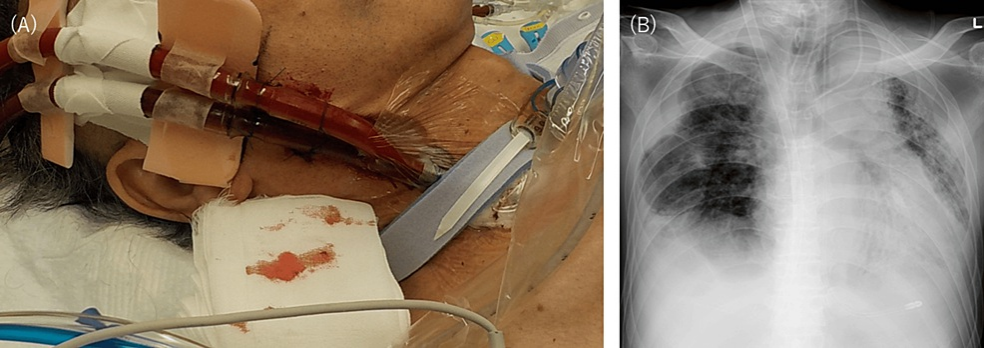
Clinical and X-ray images after the double-lumen cannula (DLC) insertion (A) Cervical image after DLC insertion taken on day 119. The DLC was firmly secured in the right neck without instability or displacement. (B) Chest X-ray after DLC insertion via supraclavicular approach on day 119.
